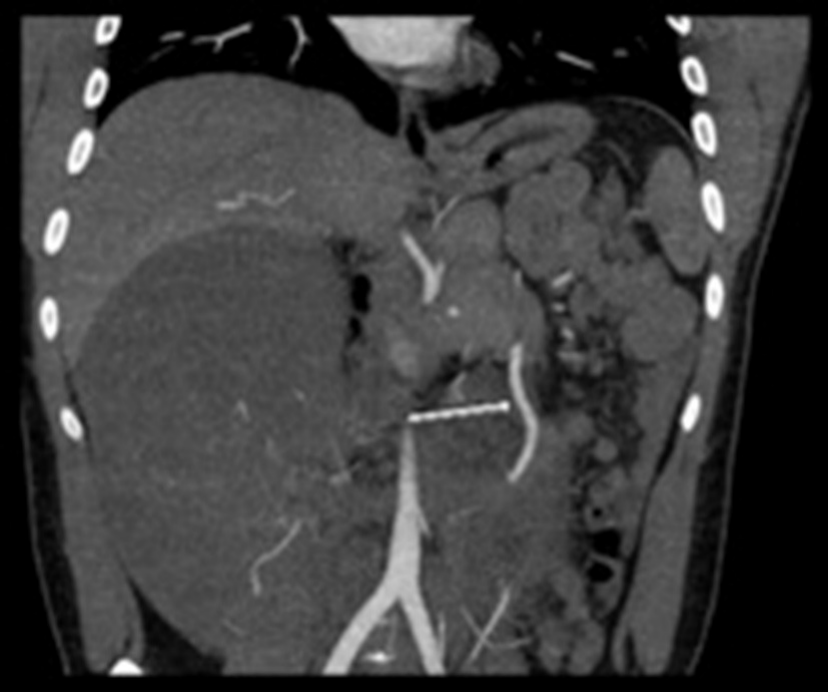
CT Angiogram arrow showing deviation of superior mesenteric artery to the left secondary to mass effect from large intra-abdominal mass. The 2nd branch of the SMA favored to represent the right colic branch courses anteriorly into and throughout the mass.
